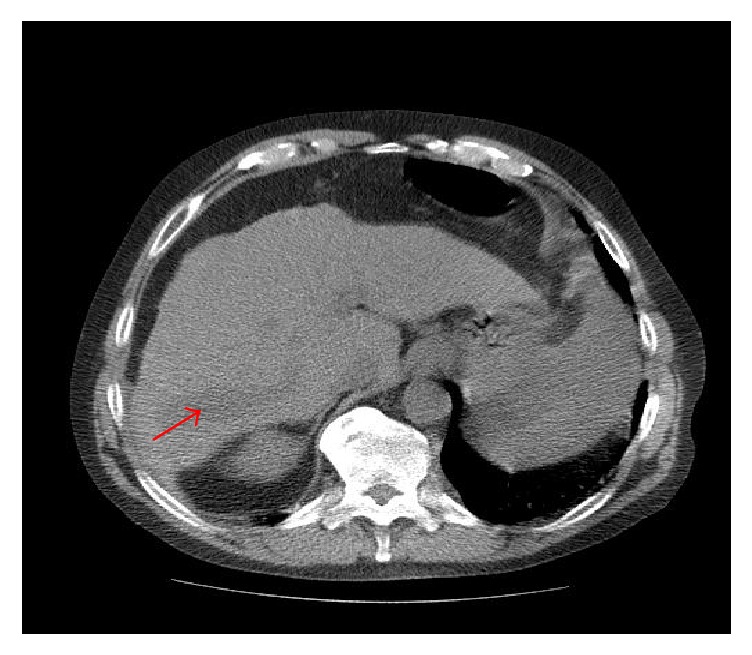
CT abdomen showing right hepatic lobe mass.
